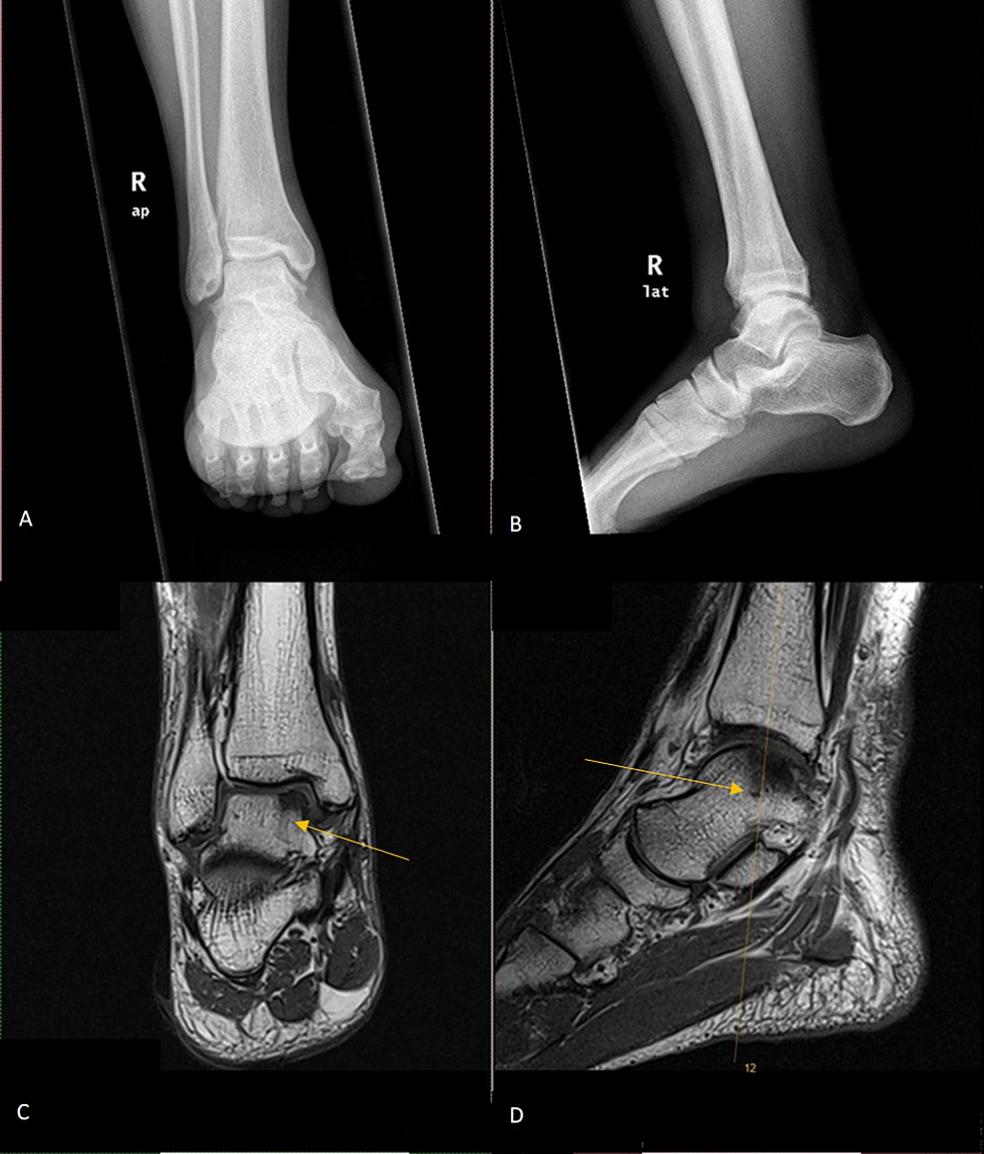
Plain radiograph of AP (A) and lateral (B) view of right ankle show fracture fragment noted in the anterior ankle joint. MRI of the right ankle (C, D) shows grade III chondromalacia (arrows) over the medial side of the talar surface with associated medial tibiotalar joint space reduction. AP: Anteroposterior
